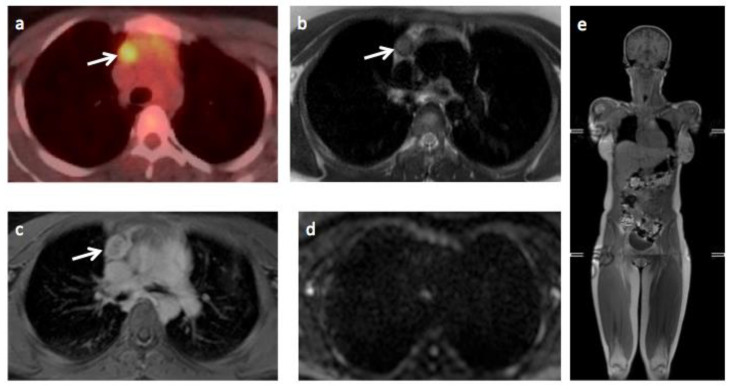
Images of a 22-year-old female patient with stage II Hodgkin’s lymphoma. Patient had left supraclavicular nodal disease (not shown here) and mediastinal disease. (a) Fused ^18^F-FDG PET-CT DWI b_1000_ (b) T2-TSE and (c) contrast-enhanced water-only mDixon showing the mediastinal disease (arrows). Mediastinal disease was not evident in (d) DWI b_1000_ and (e) pre-contrast in-phase mDixon resulting in under-staging of disease.
